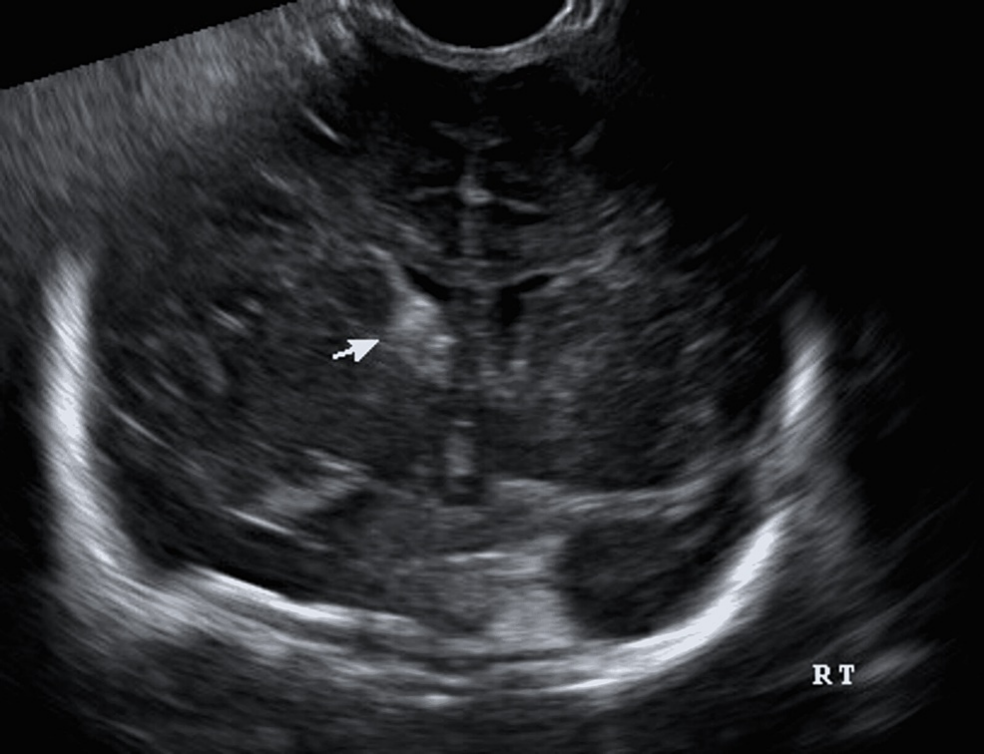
Neurosonogram shows Germinal matrix hemorrhage. Credit: Image taken by authors at the hospital.
